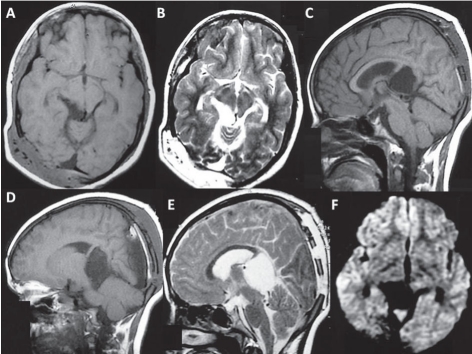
Patient 2: A: axial T1; B: axial T2; C, D: sagittal T1; E: sagittal T2; and F: diffusion-weighted axial MR images showing the postoperative tumor cavity after total excision of the lesion.
